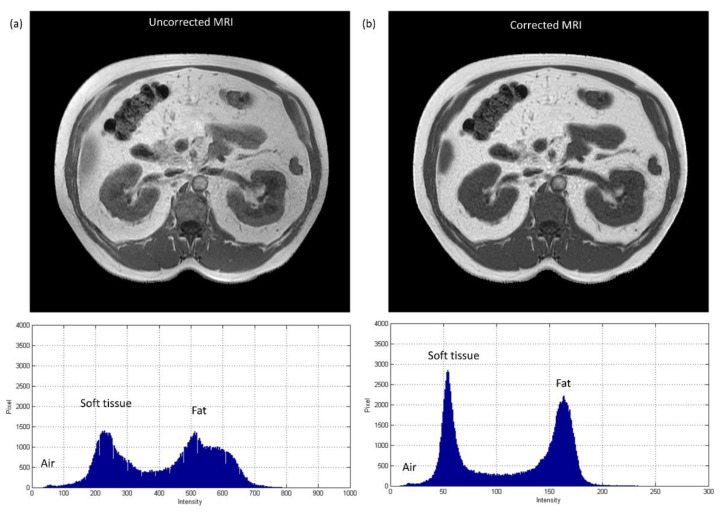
Axial image and histogram of (a) uncorrected MRI (WL/WW = 375/750) and (b) corrected MRI (WL/WW = 125/250) from 1.5 T scanner for abdominal imaging belonging to CNN testing dataset (${\mathrm{IMG}}_{\mathrm{testing}}^{\mathrm{abdomen}}$).
